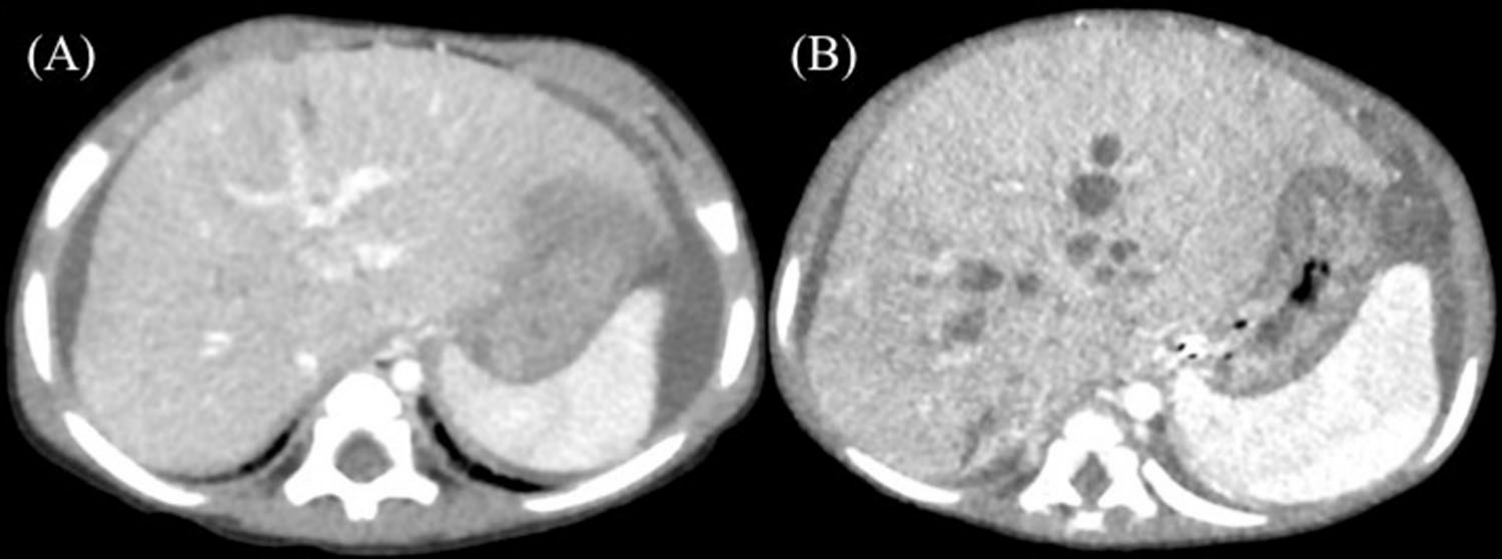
Enhanced computed tomography after portoenterostomy. (A) Before intestinal obstruction. (B) After intestinal obstruction. Multiple bile lakes suddenly appear throughout the liver
